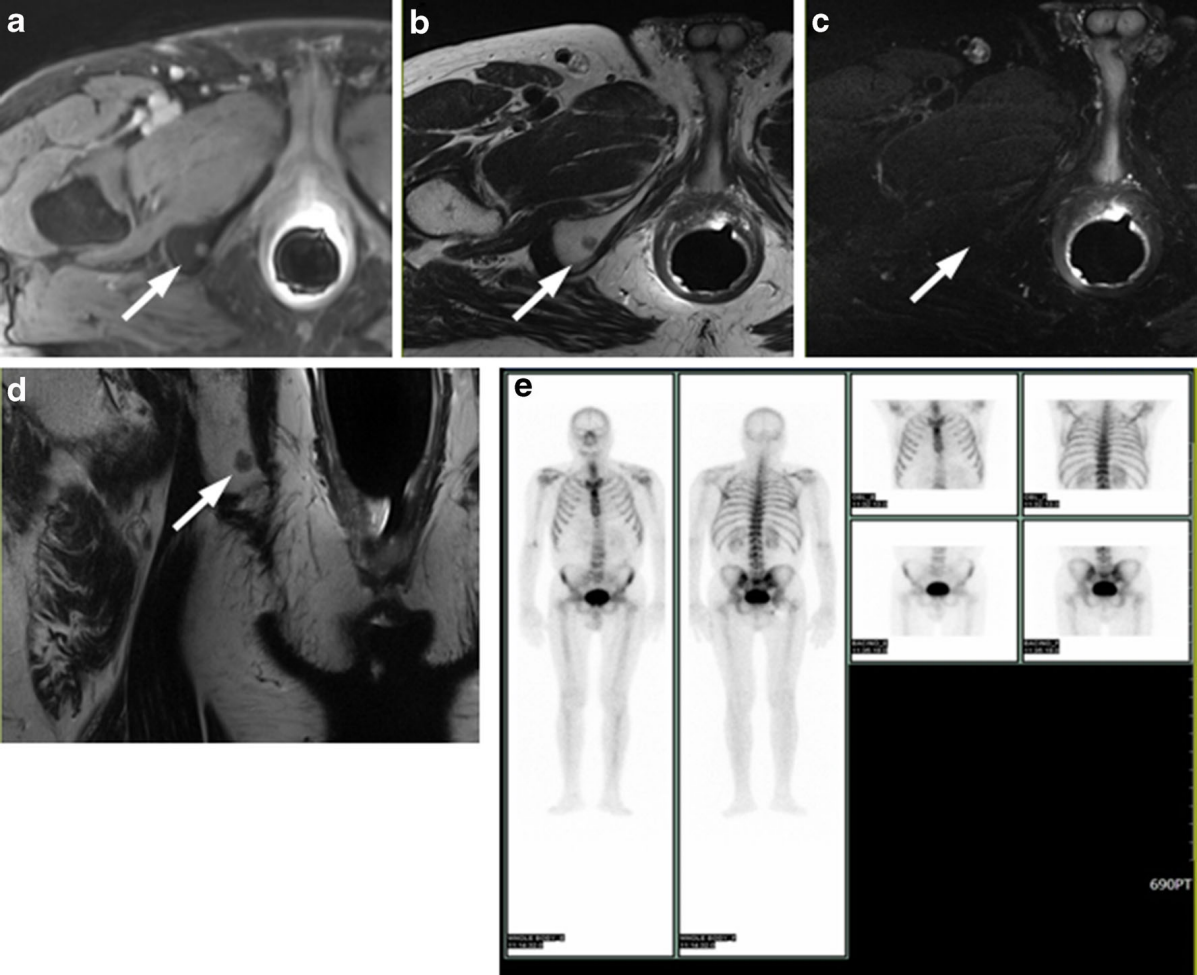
a Axial post-contrast T1-weighted fat saturated 3D FLASH (VIBE) axial image showing a small enhancing area in the right ischiopubic ramus. b Axial T2-weighted fast spin-echo (6330/115) and d coronal T2-weighted (5230/114) images display a small round hypointense area, which appears slightly hyperintense (*white arrows*) on c axial T2-weighted fat saturated image (8450/115). e Bone scan showing uptake of radiotracer in the right ischiopubic ramus. All these findings are consistent with bone metastasis. In addition, the reference standard was the drop of PSA value after hormonotherapy (from 18 ng/mL to 4.5 ng/mL)
